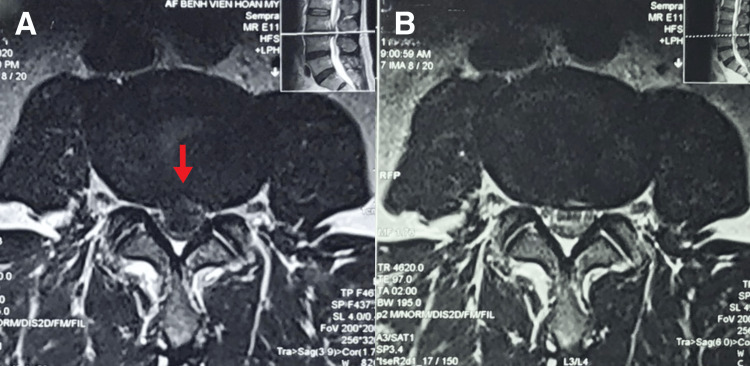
T2 MRI of the lumbar spine in axial view (A) At L3/L4, there is a severe-sized central disc extrusion (red arrow), causing severe spinal compression of the descending L4 nerve roots bilaterally within the central canal. The spinal canal shows severe narrowing, with significant central canal stenosis. (B) Nine-month follow-up examination, the image showed a total resolution of L3/L4 disc extrusion.
